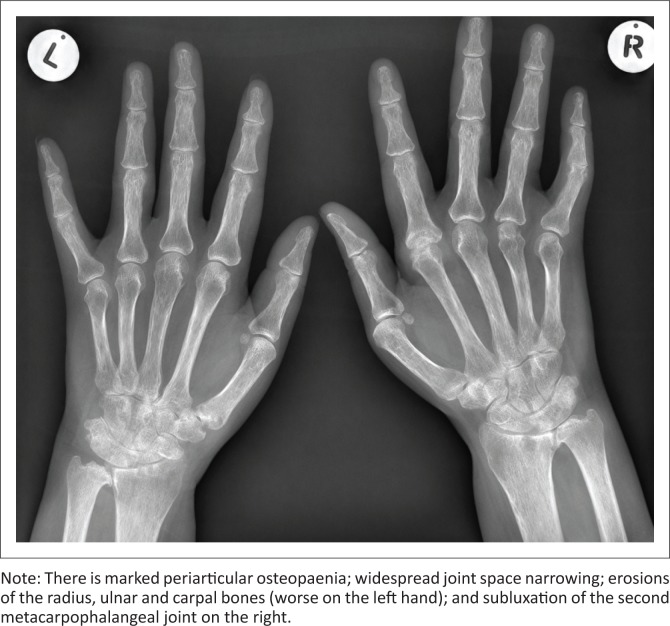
Fontal radiograph of both hands demonstrating bilateral symmetrical disease.
